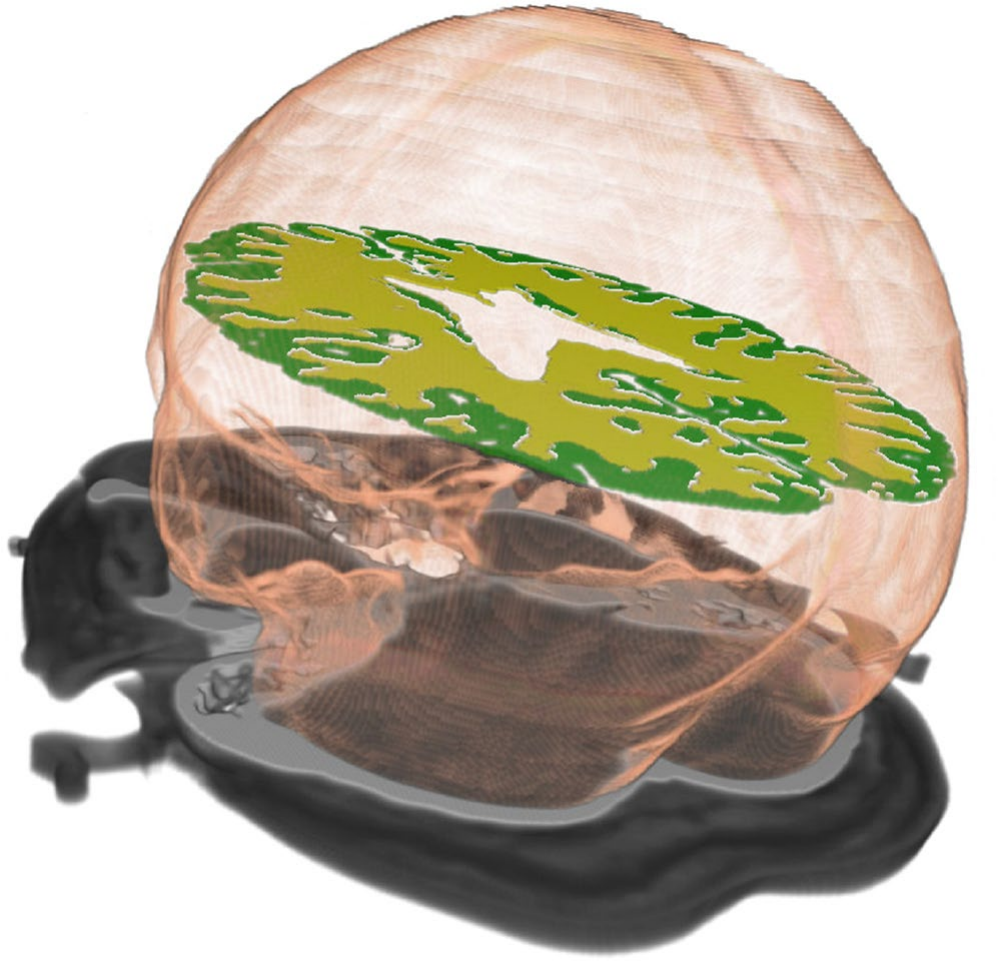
Volume rendering of the intracranial, WM and GM segmentations in 4D CT.
